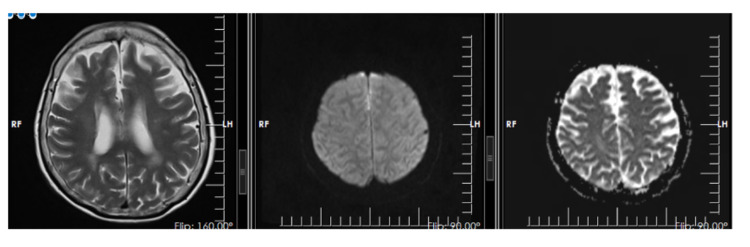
Cranial MRI done on the 22nd hospital day showing very peculiar-appearing signal abnormalities demonstrating restricted diffusion involving the interhemispheric region and the anterior frontal convexities of uncertain etiology, but are suspected to represent secondarily infected fluid collections.
